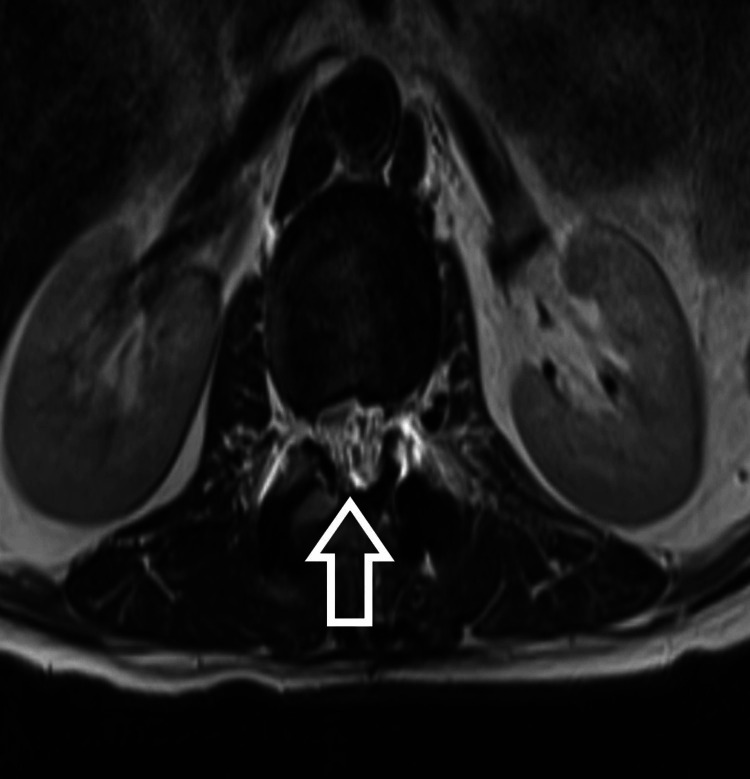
Axial MRI after 2.5 years
